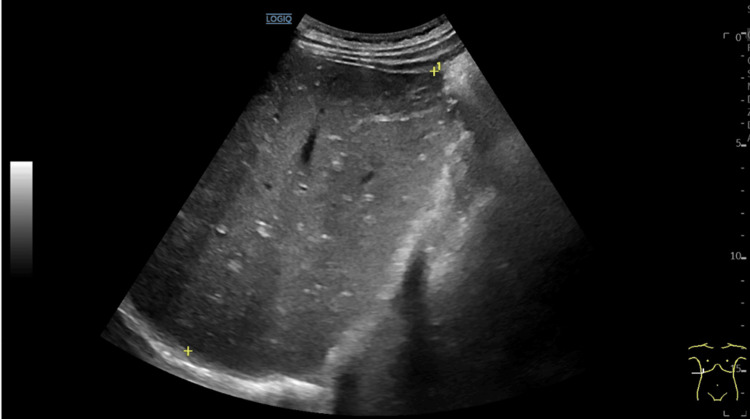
Abdominal ultrasound (A). Liver with elongated morphology (16.5 cm), regular contours, and homogeneous parenchyma. Thin-walled gallbladder, without lithiasis. No dilatation of the bile ducts. The yellow asterisks represent the longitudinal length of the liver.
